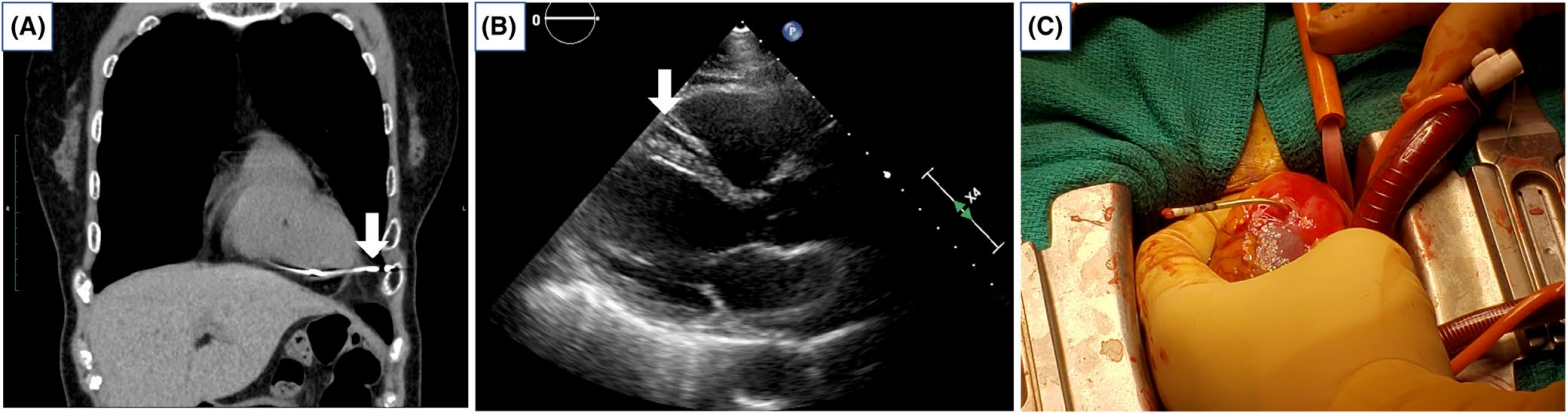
(A) Chest computed tomography image showing the apically sited right ventricular lead perforating through the right ventricle (arrow). (B) Echocardiogram revealed no findings of cardiac tamponade. The lead staying in the right ventricle(arrow). (C) RV lead had perfectly perforated the right ventricular wall about 3 cm
